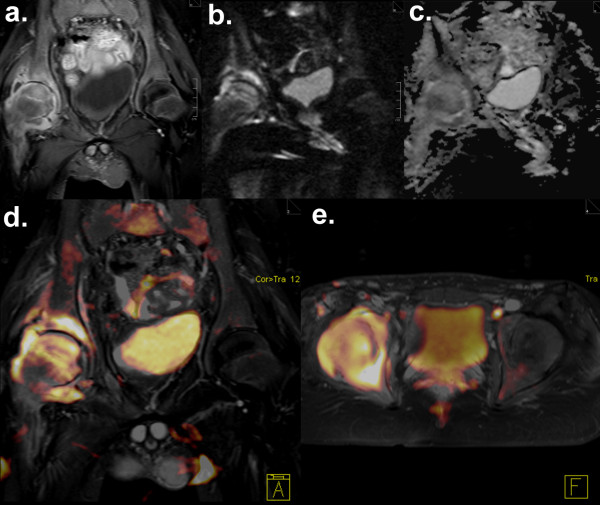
Bone marrow oedema and soft tissue oedema in tuberculous coxarthritis. Confirmed tuberculous coxarthritis of the right hip in a 15-year-old boy. Post-contrast T1W TSE FS shows severe coxarthritis (a) with osteomyelitis of the acetabulum and the femoral head and soft-tissue oedema in the right gluteus muscle. No pathological signal changes are seen in the left hip joint. Moderate distortion artefacts are noticeable on DWI with primary coronal acquisition (b), while signal changes corresponding to (a) remain clearly visible. ADC values of the inflammatory osseous and soft-tissue lesions measured on the ADC map (c) ranged between 1.60 and 1.71 × 10^-3^ mm^2^/s. Image fusion of colourised DWI b = 800 data acquired with standard transversal DWI was performed with coronal T2W TIRM (d) and transversal post-contrast T1W imaging (e).
